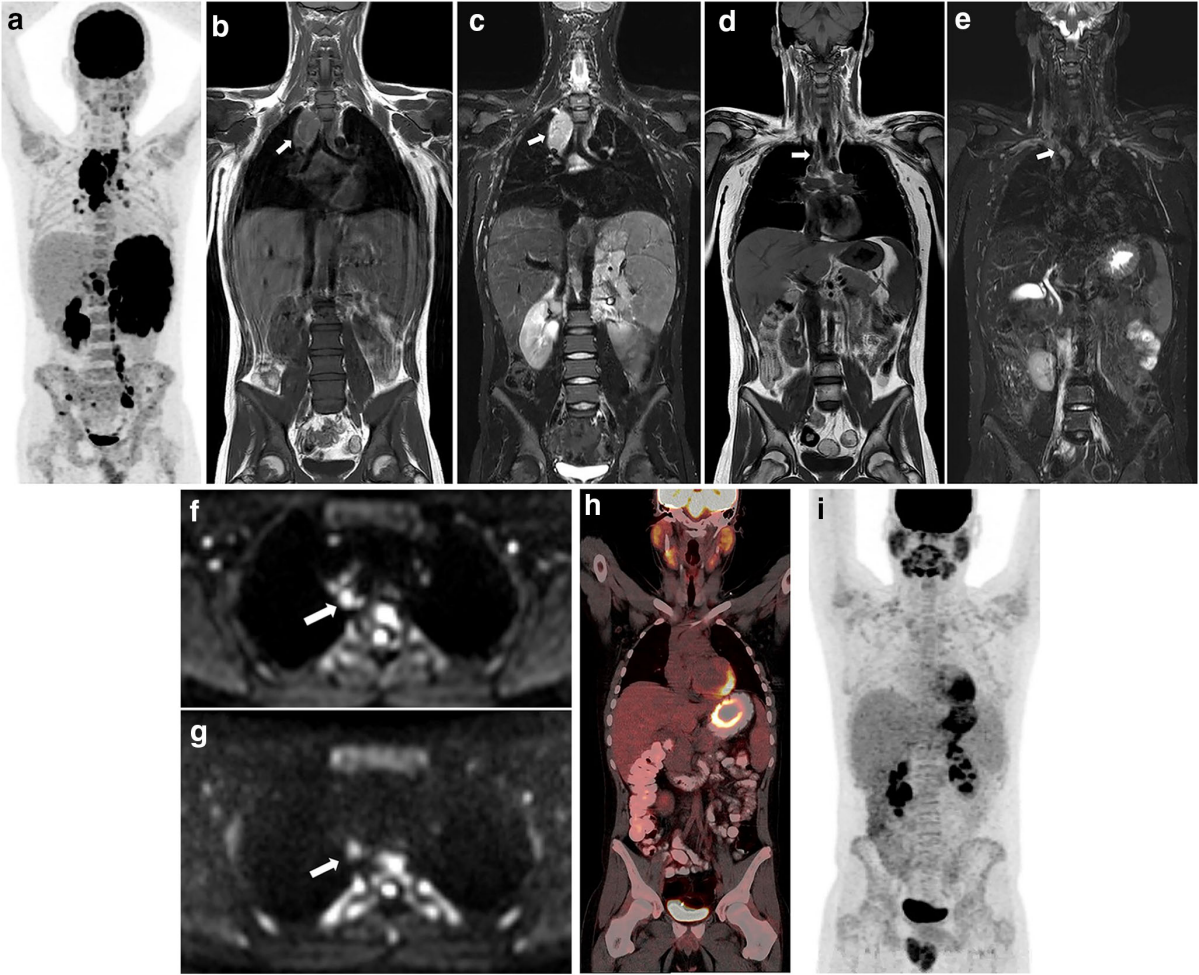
Example of an intrinsic WB-MRI error in early response assessment (ERA) after two cycles of chemotherapy. A 13-year-old boy initially diagnosed with stage III Hodgkin’s lymphoma. Maximum intensity projection (MIP) of the [^18^F]FDG-PET/CT at staging (a) shows several affected lymph node stations, including the spleen. T1-weighted (b) and T2-weighted (c) MRI at staging involvement of the mediastinum (arrows) was found. At ERA, coronal T1-weighted MRI (d) and T2-weighted MRI (e) show the mediastinal residual lesion (arrows). At axial DWI (b100 (f), b800 (g)), restricted diffusion was seen; the mediastinal station was therefore scored positive for disease presence (arrows). At coronal [^18^F]FDG-PET/CT (h), no elevated [^18^F]FDG uptake was seen in de mediastinum. Thus, the mediastinal lesion was scored positive for residual disease at ERA WB-MRI whereas [^18^F]FDG-PET/CT showed complete response. The [^18^F]FDG-PET/CT MIP (i) shows no elevated [^18^F]FDG uptake throughout the body, indicating a complete response
